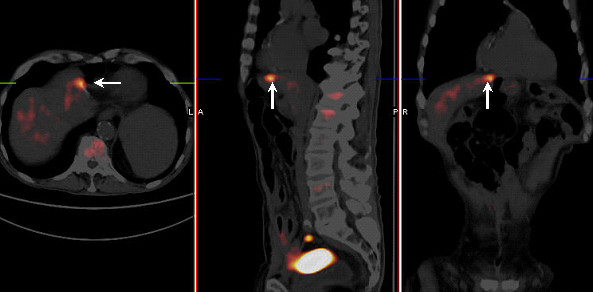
Transaxial, sagittal, and coronal PET/CT fusion images in a patient with prior left lobectomy. PET scan demonstrated a focal hypermetabolic activity within the liver at resectin margin with SUV_maxof _3.1 (arrow). Pathology demonstrated focal foreign body reaction with necrosis, organizing inflammation and fibrosis secondary to prior surgery.
